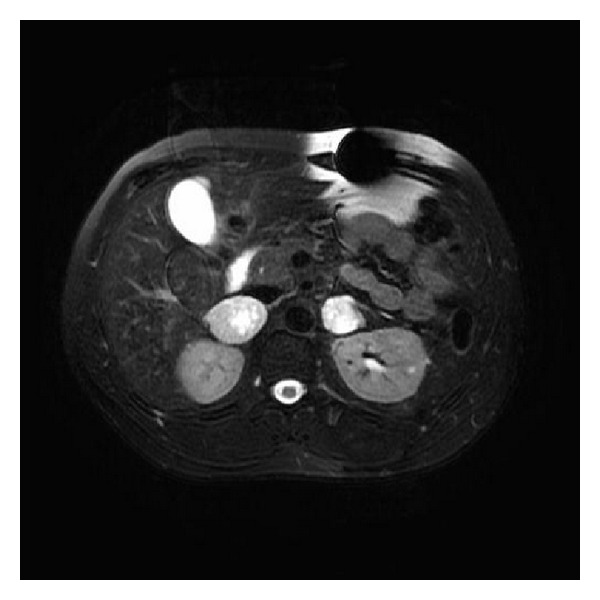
T2-weighted MRI of the abdomen showing bilateral adrenal masses. The left mass measures 4.3 cm and the right mass measures 2.8 cm in greatest dimension.
